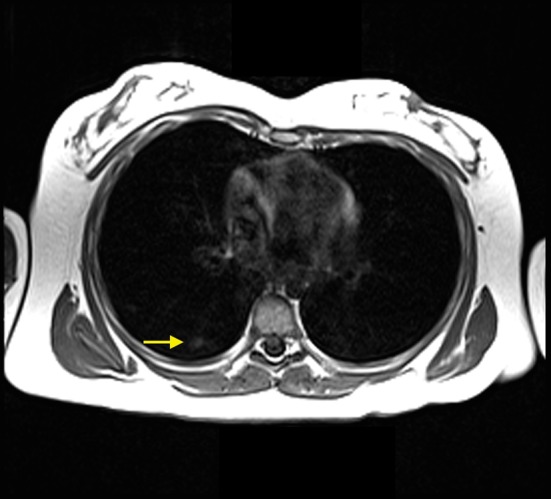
MRI scan obtained November 2012. Axial T1 image showed multiple small peripheral airspace opacities in the right lower lobe (yellow arrow), which was compatible with alveolar hemorrhage given the patient’s history and clinical presentation.
